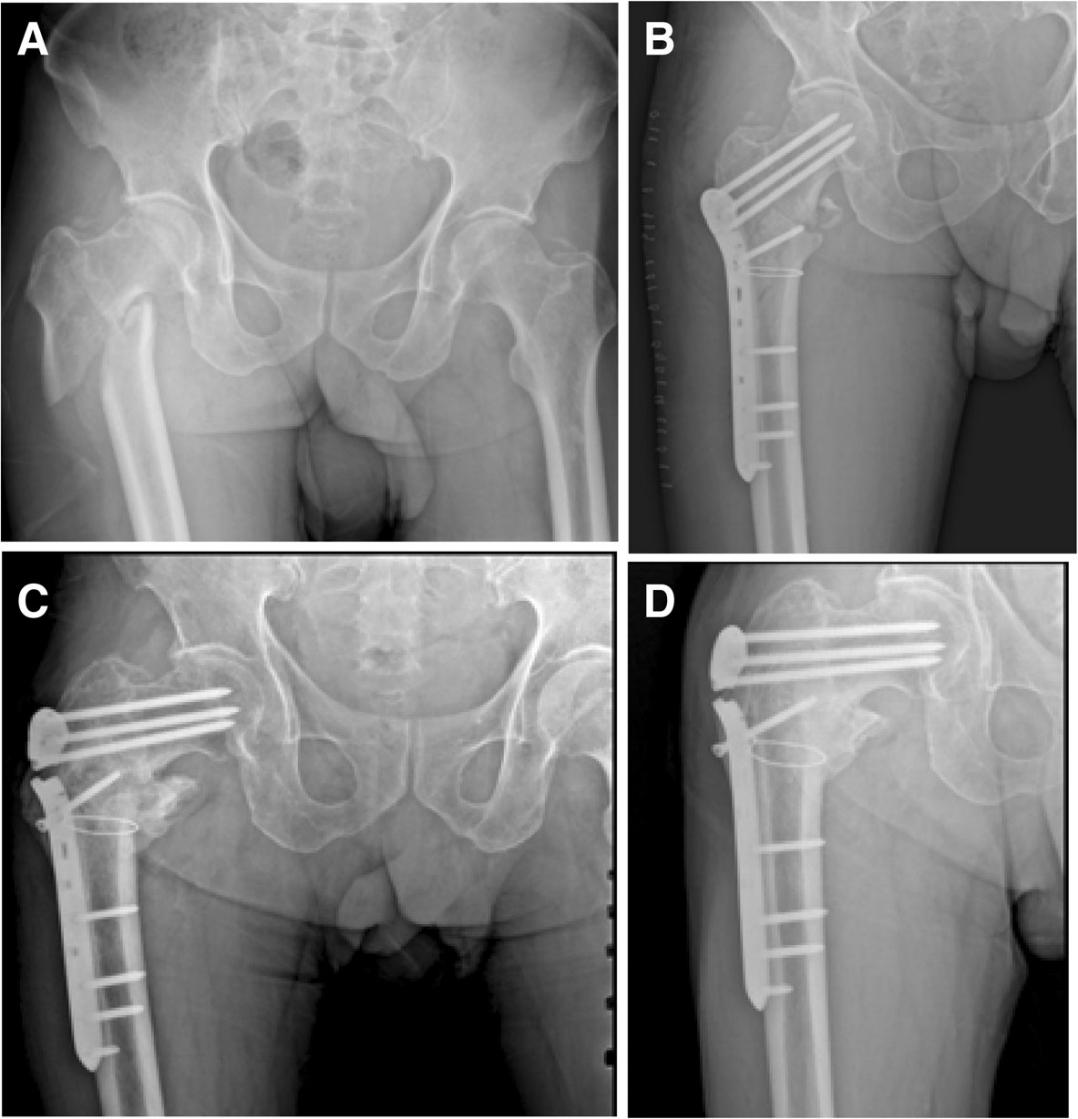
A 71-year-old male with AO/OTA type 31A3.3 experienced plate breakage. a Radiograph after injury. b Postoperative radiograph showing good reduction and fixation with PFLP (1 week after surgery). c Plate breakage and varus collapse 36 weeks after surgery. d Seventy-two weeks postoperative radiograph showing malunion and varus deformity
